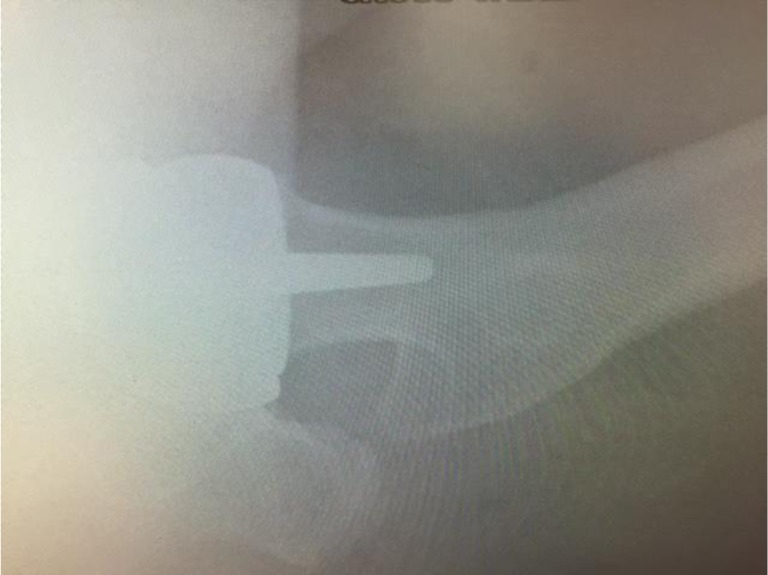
Post-operative lateral plain film radiograph of the patient’s left hip. No cause of the patient’s mechanical symptoms was detected
